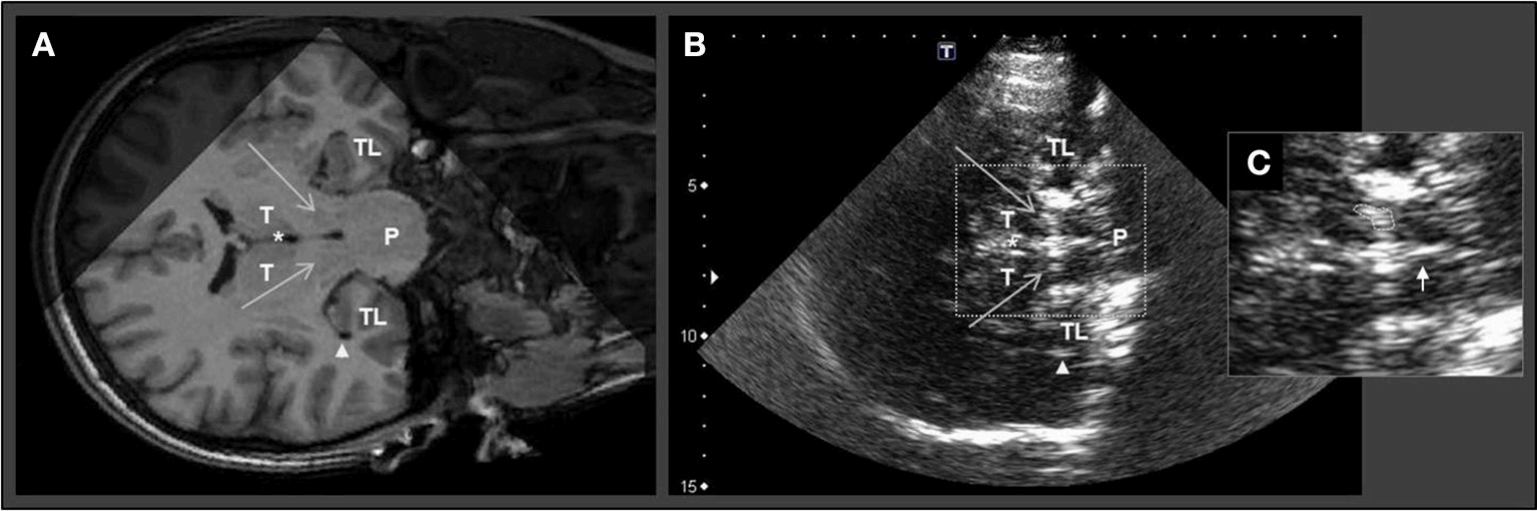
Corresponding MRI and TCS images of coronal examination planes in a patient with idiopathic Parkinson's disease. Coronal MR scanning plane (A) and corresponding TCS examination plane (B) at brainstem level. (C) Zoom in of midbrain structures for planimetric assessment of the echogenic area of substantia nigra and evaluation of brainstem raphe. The large arrows in (A) and (B) indicate substantia nigra (note that in TCS the SN is displayed echogenic). White asterisks mark the third ventricle. Arrowheads in (A) and (B) indicate the inferior horn of lateral ventricle. Small arrow in (C) indicates the normal appearing brainstem raphe. TL, temporal lobe; P, Pons; T, thalamus.
